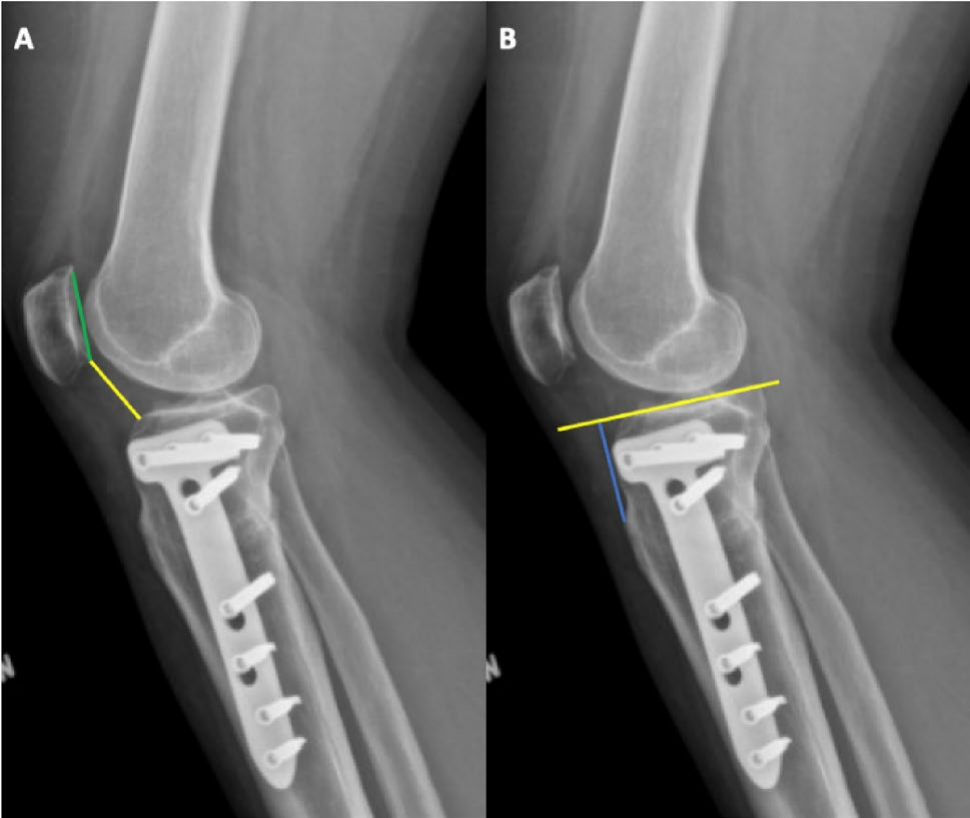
Radiographic measurement of patellar height and joint line position. A Caton–Deschamps Index (CDI): Defined as the ratio between the distance from the inferior pole of the patella to the anterosuperior aspect of the tibial plateau (yellow line) and the length of the patellar articular surface (green line). B Tibial tubercle–joint line (TT–JL) distance: Measured as the perpendicular distance (blue line) from a line along the tibial plateau surface (yellow line) to the most prominent point of the tibial tubercle
